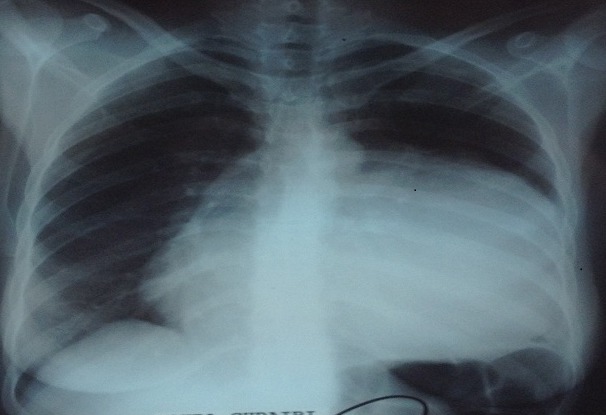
La radiographie du thorax montrant une opacité de tonalité hydrique occupant la moitié inférieure de l'hémi-champ pulmonaire gauche à bord externe en contacte avec la paroi, et à bord interne noyé dans le médiastin
